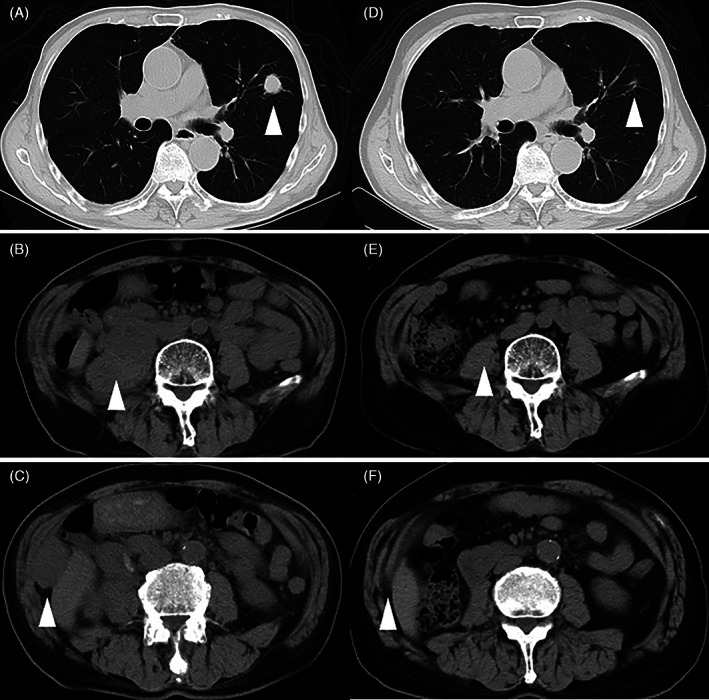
Computed tomography (CT) taken before (A–C) and after the administration of nivolumab (D–F) (arrows). (A–C) CT shows lung metastasis (A), local recurrence (B), and peritonitis carcinomatosis (C). (D–F) After 24 months of nivolumab treatment, the patient achieved a complete response with multiple metastases on CT (arrows)
